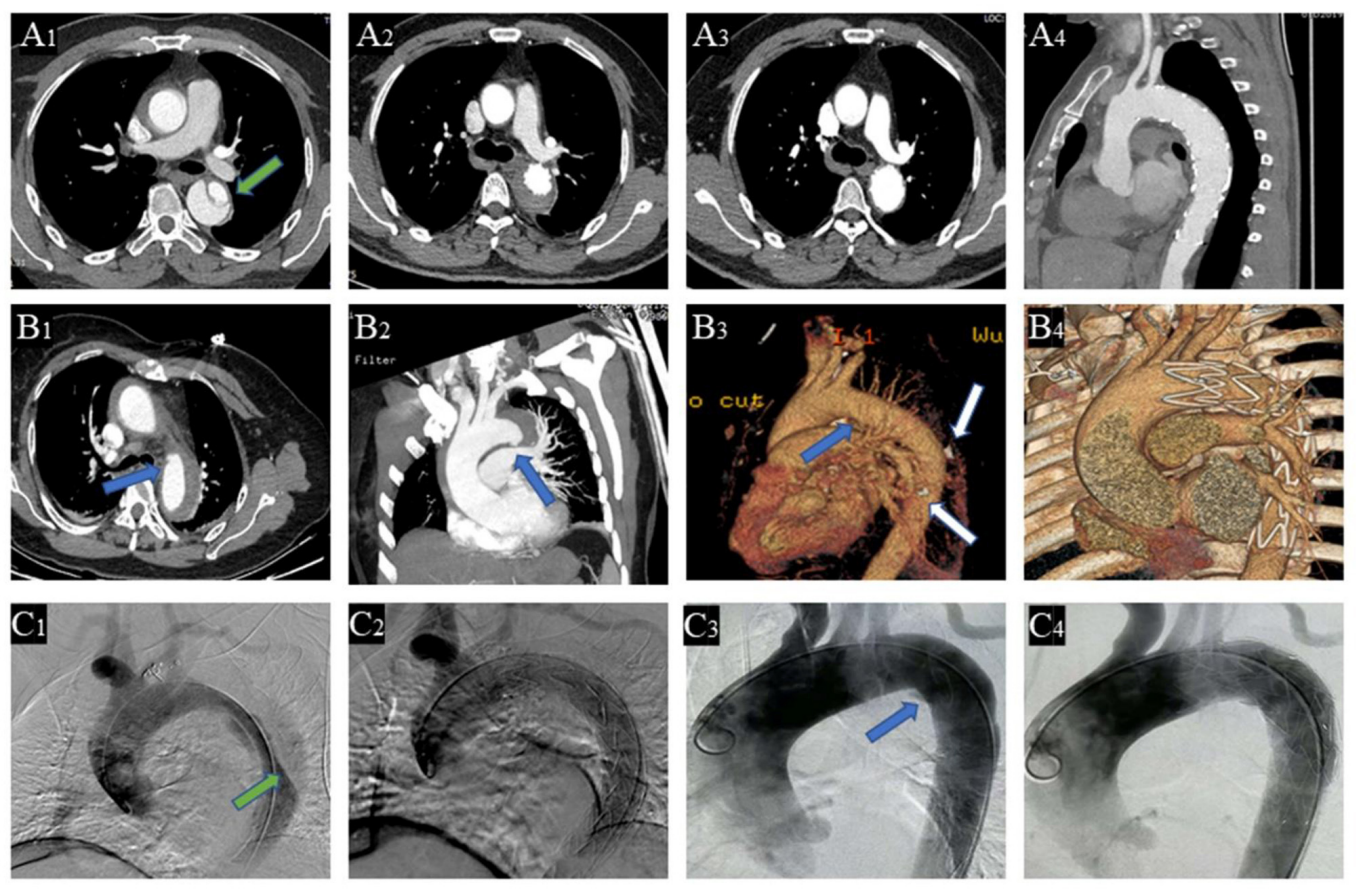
Contrast-enhanced computed tomography angiography (CTA) of the patients with retrograde type A aortic intramural hematoma (TAIMH). A patient with entry tear (green arrow) in descending aorta was treated by thoracic endovascular aortic repair (TEVAR), and the image was collected at admission (A_1_), 2 weeks after TEVAR (A_2_), and 3 months after TEVAR (A_3,4_), respectively. Another patient with penetrating atherosclerotic ulcer (PAU, blue arrow) and calcification plaque (white arrow) at admission (B_1−3_) and 2 weeks after TEVAR (B_4_), respectively. Intraoperative angiography of the two patients with retrograde TAIMH before (C_1_, entry tear, green arrow; C_3_, PAU, blue arrow) and after implanting stent grafts (C_2_, entry tear was blocked; C_4_, PAU was blocked).
